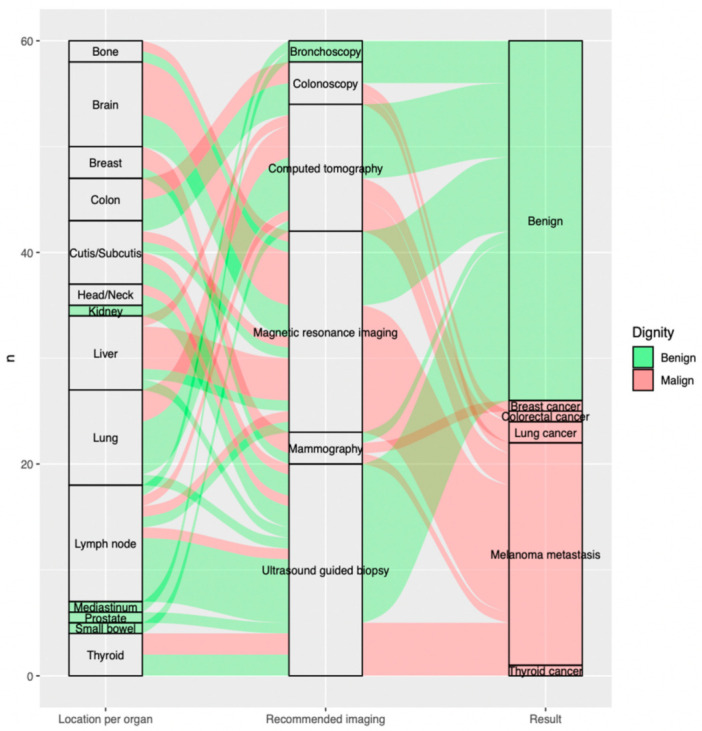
Sankey plot showing, per included indeterminate finding on the FDG-PET/CT (*n* = 60), on the far left, (a) location per organ; in the middle, (b) recommended imaging for further evaluation; and the far right, (c) outcome of additional imaging. Benign findings are displayed in green, malign findings in red arrows. (a) Location per organ (on the left from the bottom to the top): thyroid (*n* = 4; 5.0%, small bowel (*n* = 1; 1.7%), prostate (*n* = 1; 1.7%), mediastinum (*n* = 1; 1.7%), lymph node (*n* = 11; 18.3%), lung (*n* = 9; 15.0%), liver (*n* = 7; 11.7%), kidney (*n* = 1; 1.7%), head/neck (*n* = 2; 3.3%), cutis/subcutis (*n* = 6; 10.0%), colon (*n* = 4; 6.7%), breast (*n* = 3; 5.0%), brain (*n* = 8; 13.3%), bone (*n* = 2; 3.3%). (b) Recommended imaging (in the middle from the bottom to the top): ultrasound guided biopsy (*n* = 20; 33.3%), mammography (*n* = 3; 5.0%), magnetic resonance imaging (*n* = 19; 31.7%), computed tomography (*n* = 12; 20.0%), colonoscopy (*n* = 4; 6.7%), bronchoscopy (*n* = 2; 3.3%). (c) Result (on the right from the bottom to the top): thyroid cancer (*n* = 1; 1.7%), melanoma metastasis (*n* = 21; 35.0%), lung cancer (*n* = 2; 3.3%), colorectal cancer (*n* = 1; 1.7%), breast cancer (*n* = 1; 1.7%), benign (*n* = 34; 56.7%).
